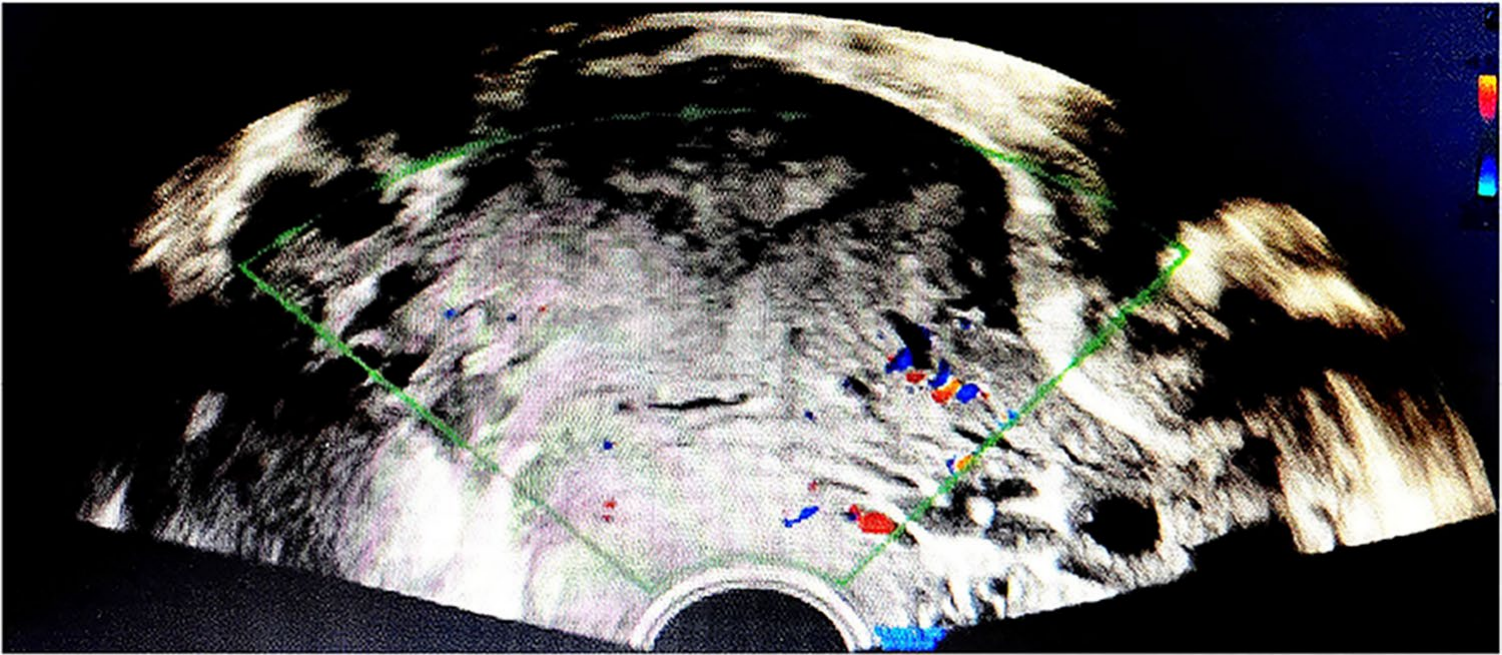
Doppler examination revealed a 1.4 × 2.0 × 0.7 cm hypoechoic area was observed at the incision, with uneven internal echo, communication with the uterine cavity, and visible blood flow signals around
